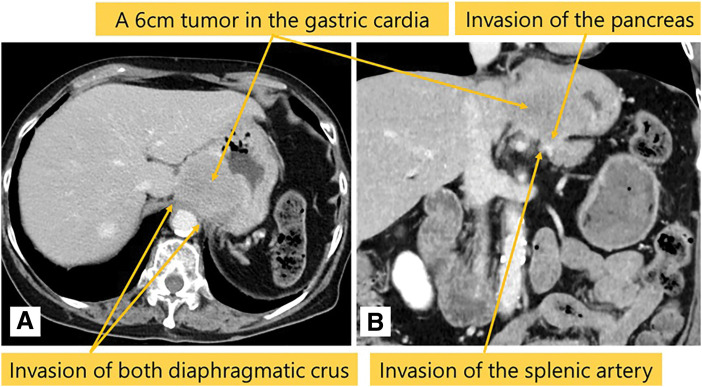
Contrast enhanced CT images. (A, B) Contrast enhanced CT revealed a 6-cm tumor in the gastric cardia with suspected invasion of the diaphragmatic crus, pancreas, and splenic artery. No other potential primary lesions were identified.
